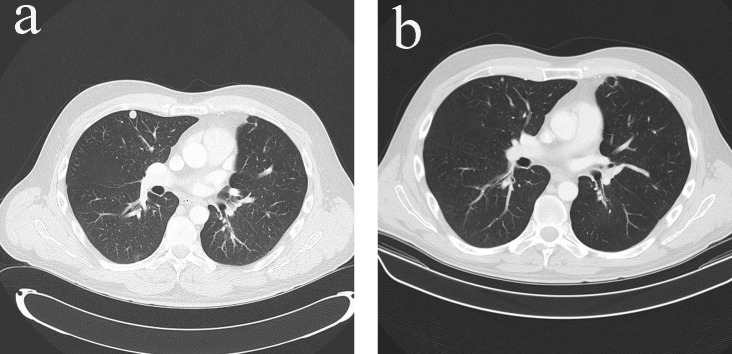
(a) Pulmonary metastases in CT scan (transverse view) before additional chemotherapy with EDP. (b) Resolution of pulmonary metastases in CT scan (transverse view) after additional chemotherapy with EDP.
